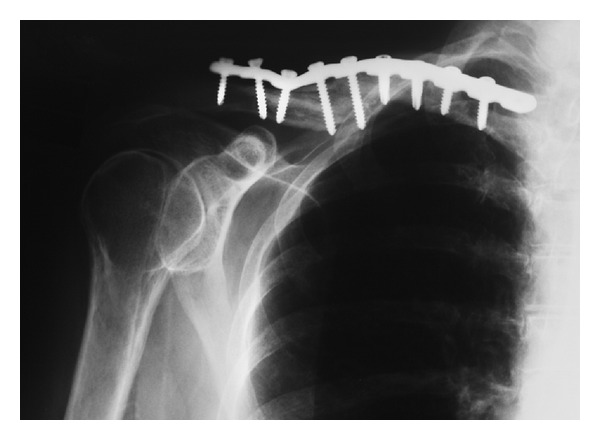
Radiograph of the right clavicle with fracture fixation plate and screws. The inferior prominence of the bone may have reaggravated the brachial plexus injury. Revision surgery was not required.
